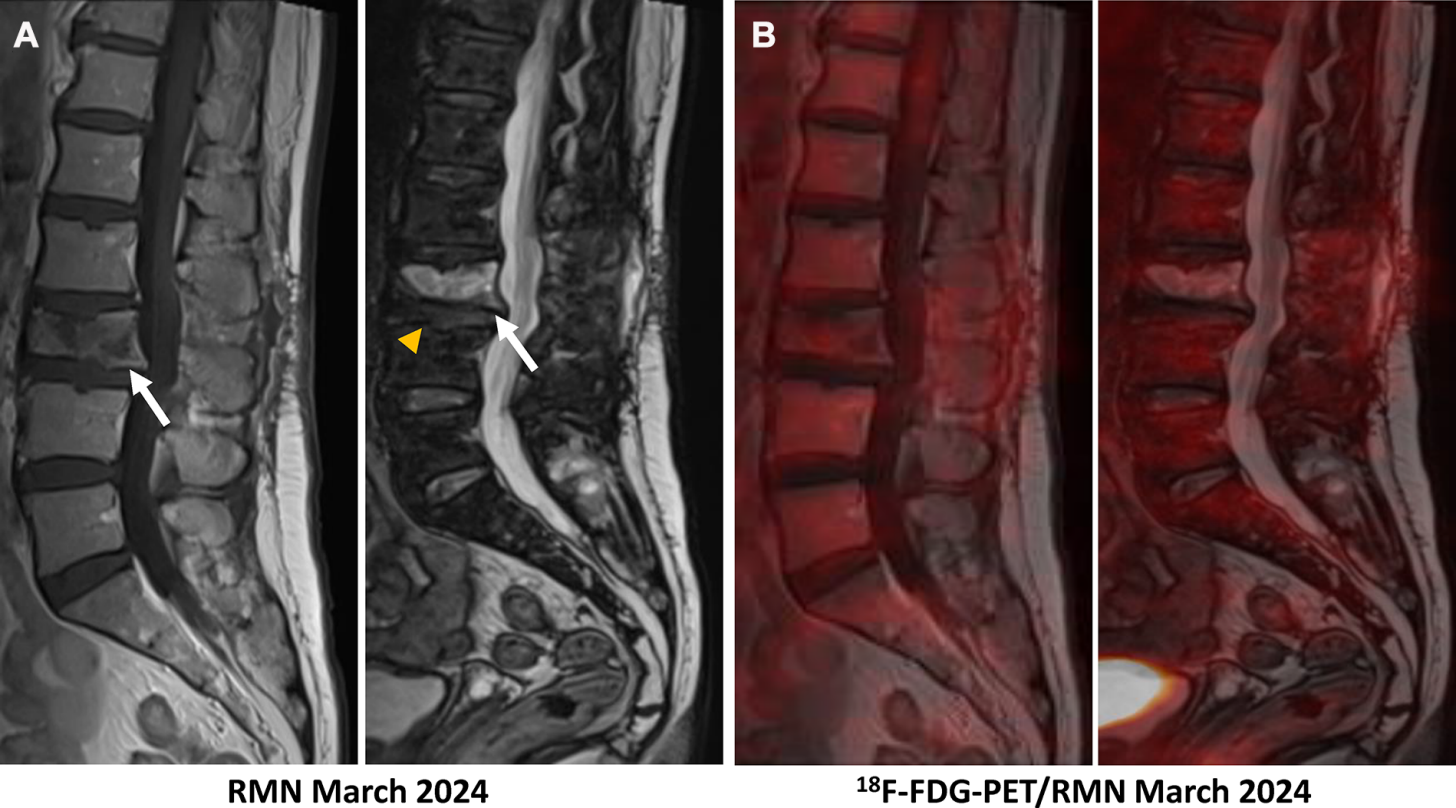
(A) The follow-up MRI realized in March 2024 showed traces of the laminectomy performed on L3, a clear reduction in tumour infiltration of the vertebral body of L3 in both phases (hypo-signal in T1 and hypersignal in T2–phase, white arrows) with pathological compaction (lost of height: 10 mm), and a reduction in the inflammatory spindle which extended anteriorly and posteriorly. The L3–L4 intervertebral disc remains reshaped but no longer shows associated enhancement (yellow head arrow). (B) A merged image of ^18^F-FDG-PET and MRI showed a complete metabolic response at the L3 vertebra and L3–L4 intervertebral disc after 4 cycles of chemotherapy.
